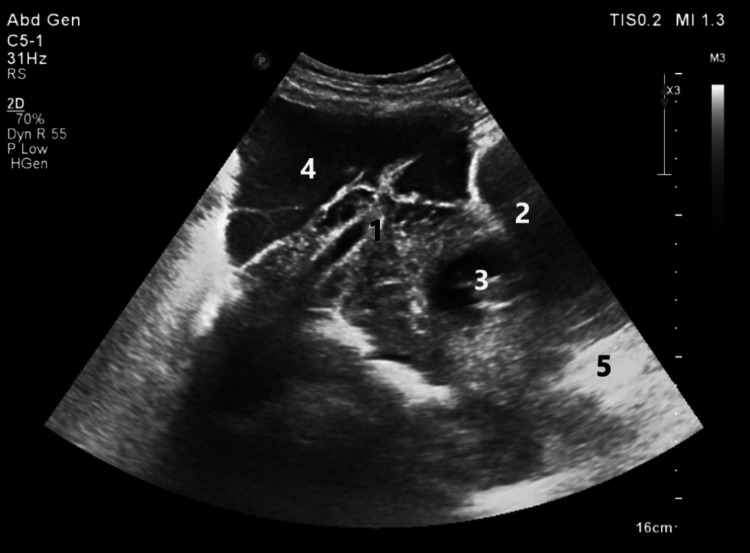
Bedside ultrasound image showing the rupture site with an ill-defined margin of myometrium and serosa of uterus fundus (1), urinary bladder (2), intrauterine viable fetus (3), pelvic free fluid with debris blood (4), and cervix (5).
